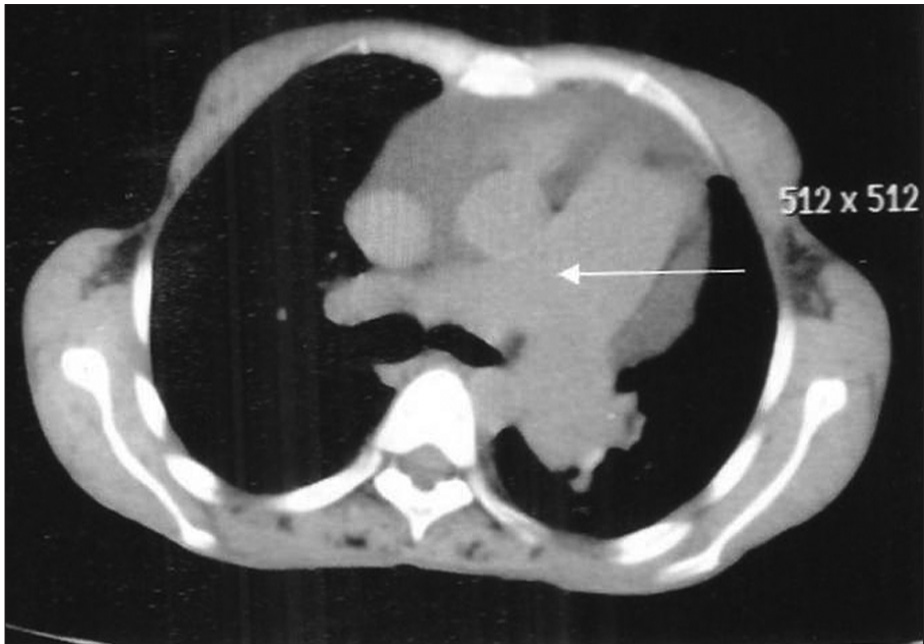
HRCT thorax showing dialated pulmonary artery
